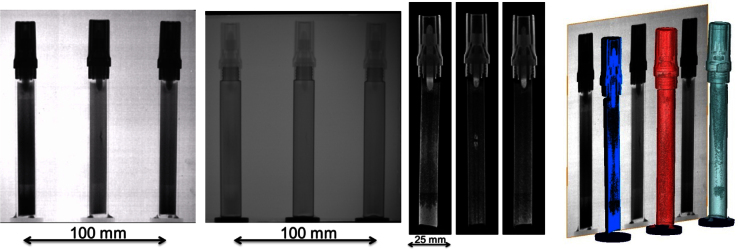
Results of the pilot experiment using the rotation axis demultiplexer (POLYTOM): Neutron radiography of three whiteboard markers (far left), corresponding X-ray radiography (left), reconstructed vertical slices from neutron tomographic datasets showing clear differences in the distribution of ink inside the whiteboard markers (right), 3D renderings of the neutron tomographic datasets showing the distribution of predominantly hydrogenous materials) in the whiteboard markers (far right).
